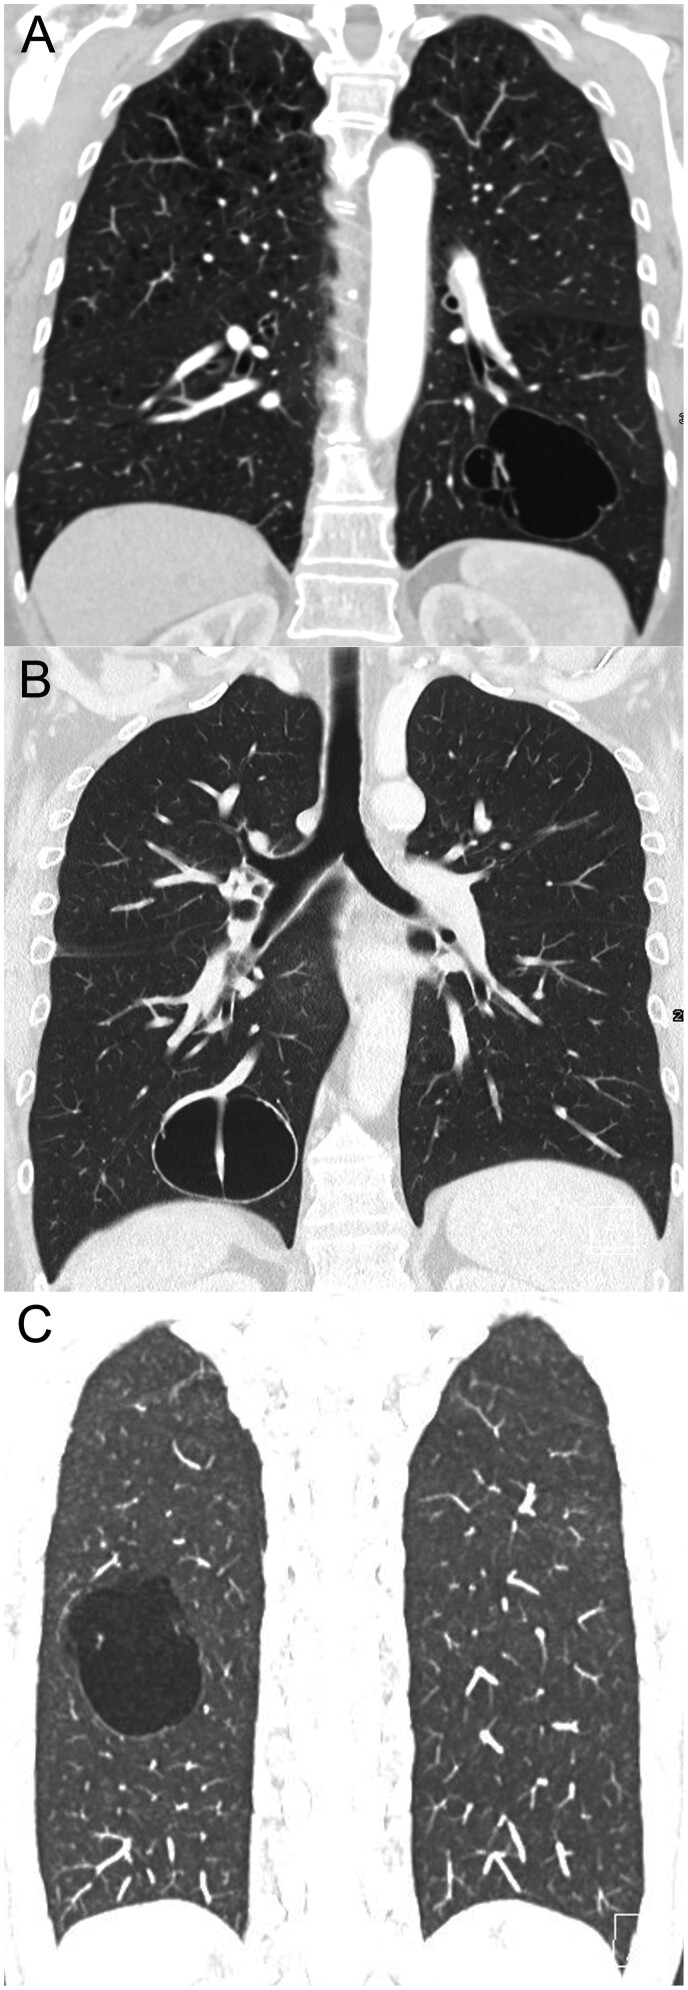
Chest computed tomography (CT) in coronal view. (A) In Case 1, contrast-enhanced CT showed a thin-walled lobulated cyst in the left lower lobe with some thin septae. There are no solid components and no mediastinal or hilar lymphadenopathy. (B) In Case 2, CT demonstrated a right lower lobe cyst with a thick wall (>4 mm). (C) In Case 3, CT showed a thin-walled (<4 mm) cyst in the right lower lobe.
